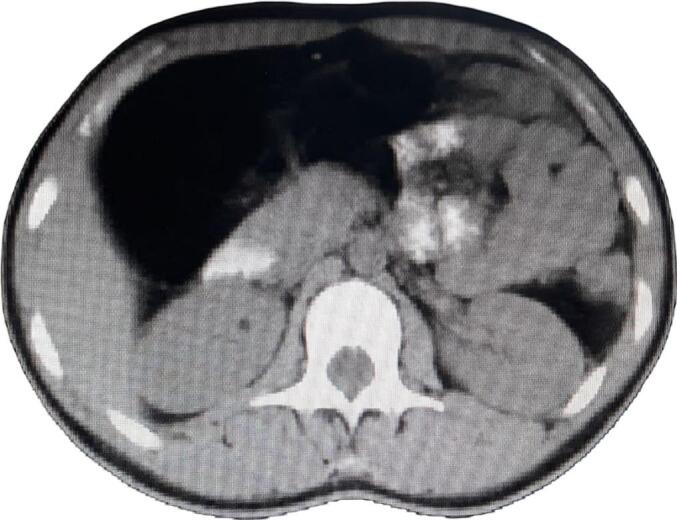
Selected cut from a contrast-enhanced (CT) scan of the abdomen revealed a well-defined, intraperitoneal, homogeneous, hypodense, non-enhancing mass located in the right upper and mid-abdomen, measuring 13.2 × 8.8 × 10.3 cm.
